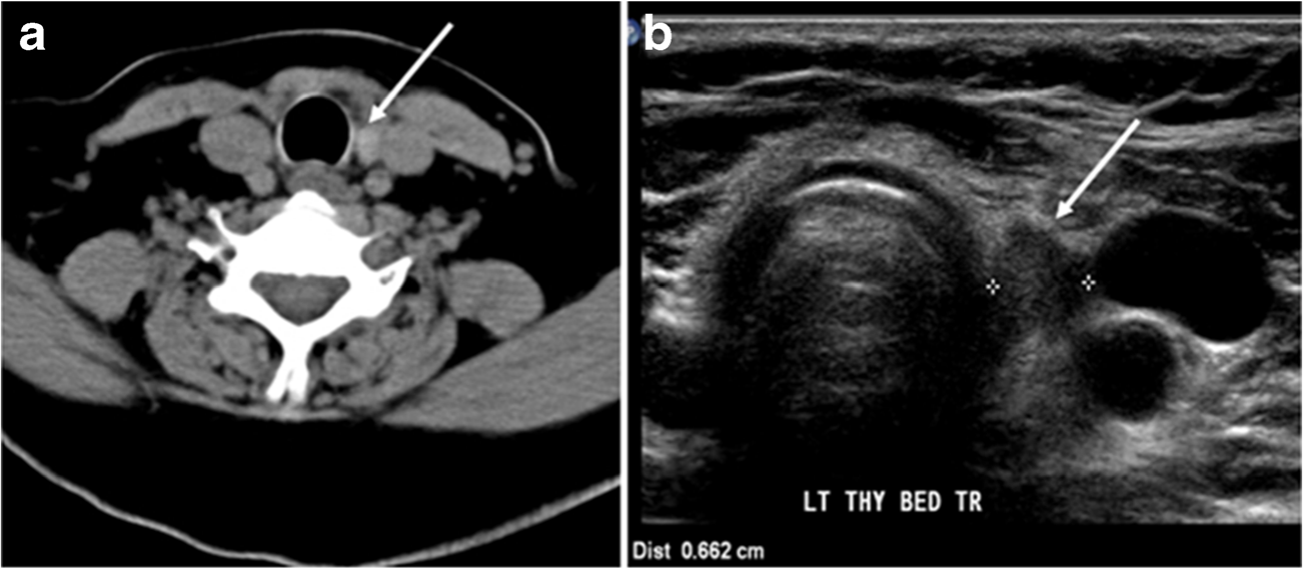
A 51-year-old female patient post total thyroidectomy for PTC with elevated thyroglobulin measurement. a Axial non-enhanced CT scan of the neck at the level of the thyroid bed demonstrates a well-defined, rounded, homogenously dense soft tissue situated between the trachea and left internal jugular vein (*white arrow*). b Transverse ultrasound image of the neck demonstrates a well-defined, homogenous, hypoechoic soft tissue nodule measuring 6 mm (*white arrow*) with no detected micro-calcifications. Biopsy showed a predominantly residual normal thyroid tissue with micro-foci of PTC
